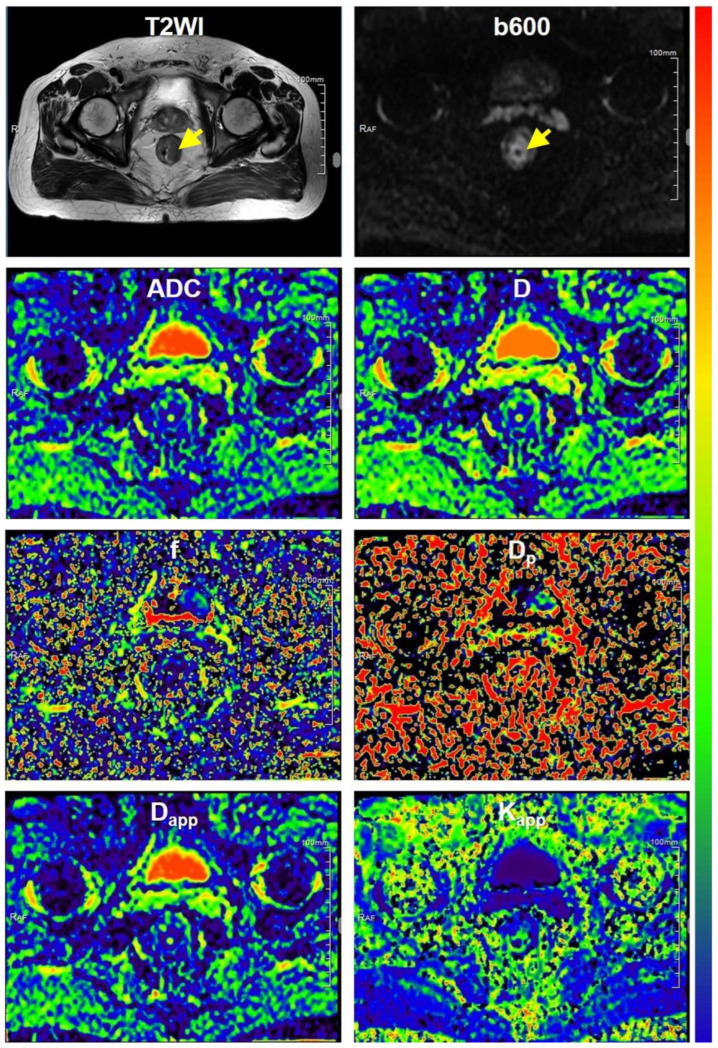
Representative MR images of a patient with low grade rectal cancer. Notes: All parametric maps employed a consistent color bar, spanning from blue to red, to signify the minimum and maximum values, respectively. The parametric ranges for ADC, D D_p_, f, D_app_, K_app_ are as follows: 0.0 to 5.0 × 10^−3^ mm^2^/s, 0.0 to 5.0 × 10^−3^ mm^2^/s, 0.0 to 80.0 × 10^−3^ mm^2^/s, 0.0 to 0.8, 0.0 to 5.0 × 10^−3^ mm^2^/s, 0.0 to 2.5, respectively. The yellow arrows indicate the tumors.
